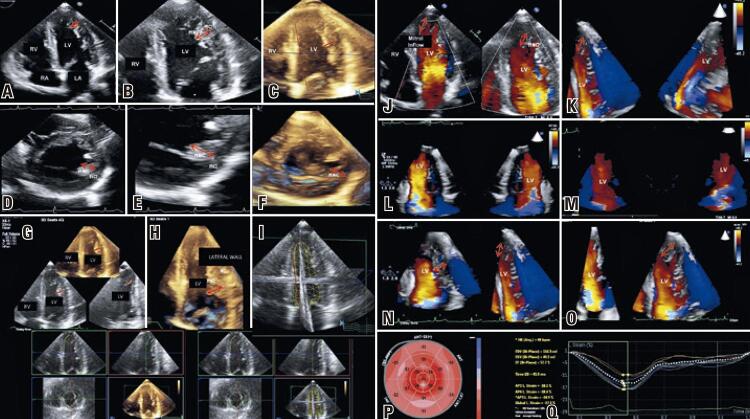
Images from conventional, three-dimensional and speckle tracking echocardiography. 2D echo and 3D echo, respectively, on apical 4-chamber view (A, B and C) and transversal view (D, E and F) depicting left ventricular noncompaction (red arrows). 3D echo on apical 4-chamber and transversal view of the same patient (C and F). 3D and triplanar views of the left ventricle (G, H and I). 2D (J) and 3D color Doppler (K to O) showing blood flow within the left ventricle recesses. Strain analysis with global longitudinal strain slightly decreased = -17% (P and Q)
